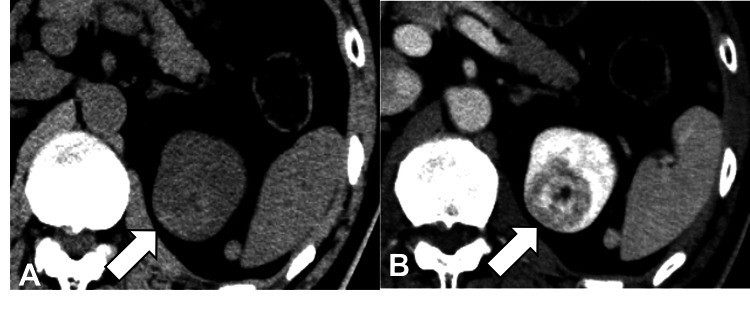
CT images of a 59-year-old man with clear cell RCC A: unenhanced image; B: enhanced image in nephrographic phase On axial UE-CT, a 2.7-cm heterogeneous isodense mass with unclear margins is seen in the left kidney (arrows). The mass protrudes slightly outside the renal cortex and its mean attenuation is 31 HU. It shows heterogeneous enhancement and proved to be a clear cell type RCC pathologically RCC: renal cell carcinoma; UE-CT: unenhanced computed tomography
